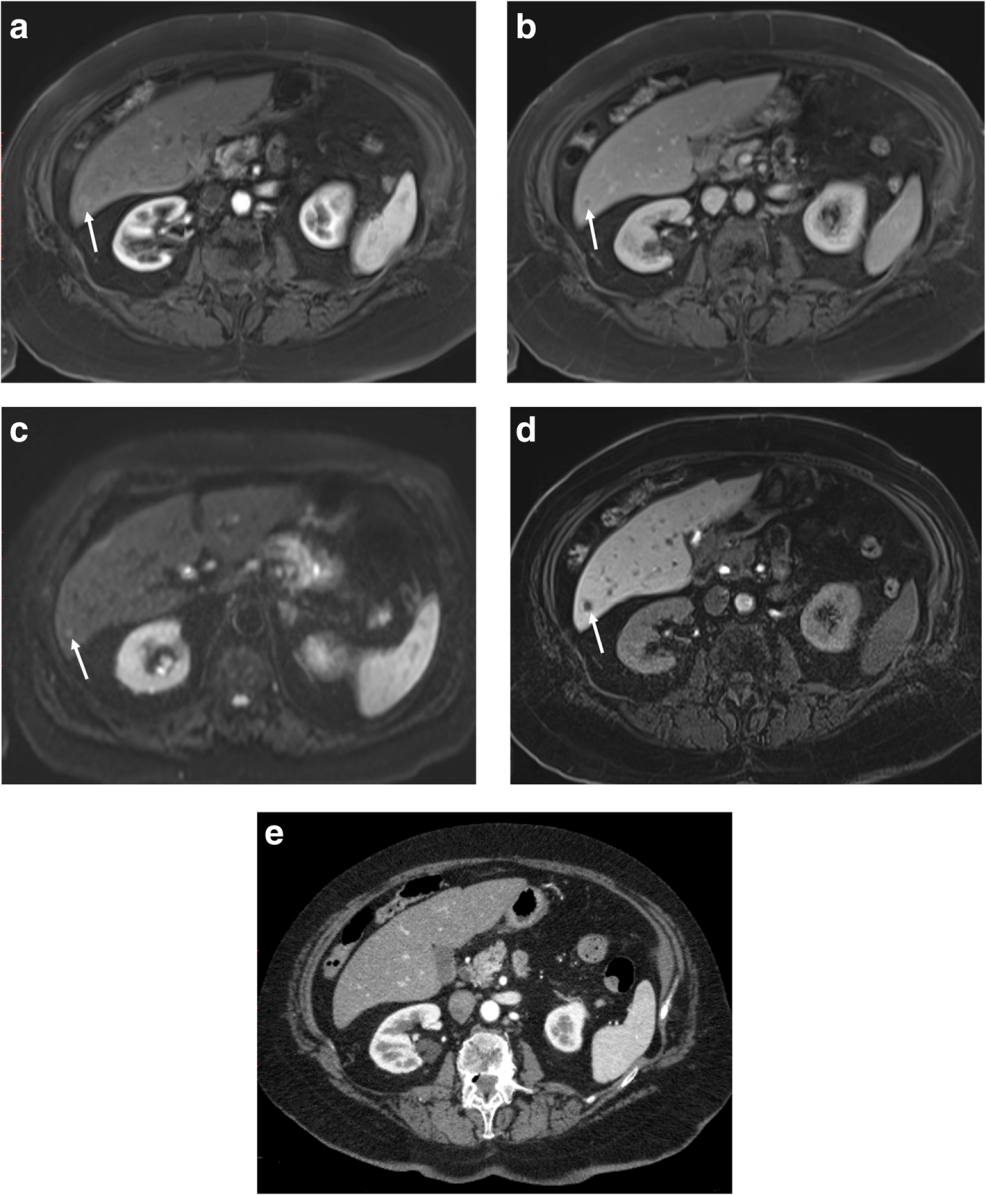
A 78-year-old female with otherwise resectable pancreatic cancer. A small liver metastasis (arrows) was diagnosed in segment 6 on EOB-MRI (a-d), but was invisible on CECT (e). While the lesion (arrows) shows faint rim enhancement in arterial (a) and portal (b) phases, and diffusion restriction in DWI (c), it is clearly detectable on hepatobiliary phase (d). This impacted the surgical plan (compared to CECT alone) with cancellation of curative-intent surgery and potential avoidance of a futile laparotomy
